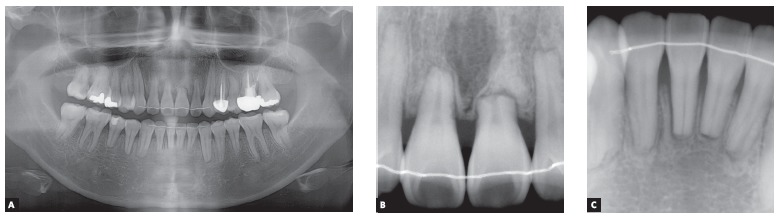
A) Post-treatment panoramic radiograph. B, C) Upper and lower incisors periapical radiographs.
